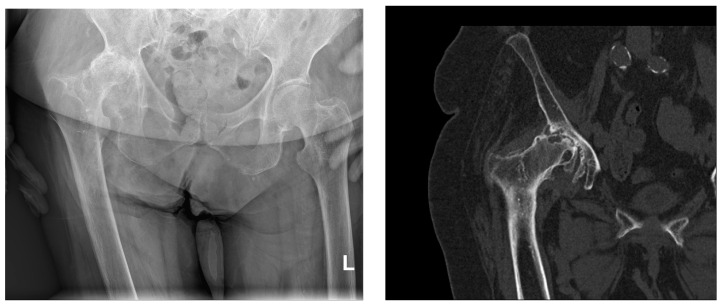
Sagittal X-ray of the pelvis and computerized tomography of the right hip (same patient). Severe right-sided coxarthrosis deformans. Grossly reduced joint space. Dense, sclerotic subchondral bone tissue of the deformed articular bodies interspersed with cyst-like transparencies of bone. Abundant marginal osteophytes of the articular surfaces and femoral head subluxation with consequent higher position of the right half of the pelvis. L—left side.
